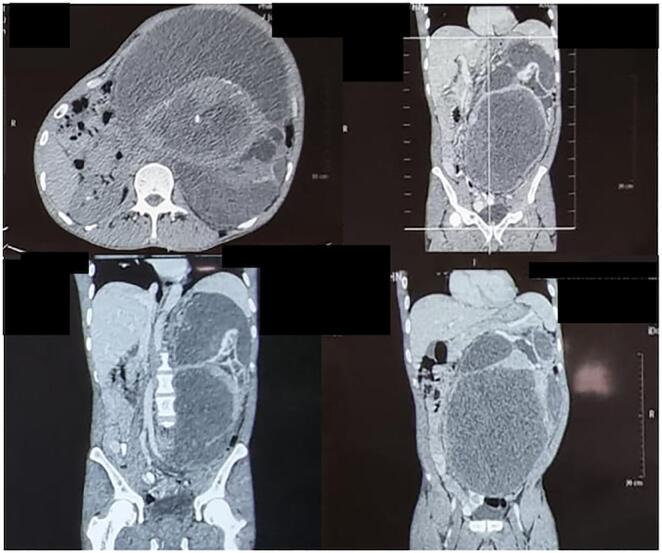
An abdominal computed tomography (CT) scan.
